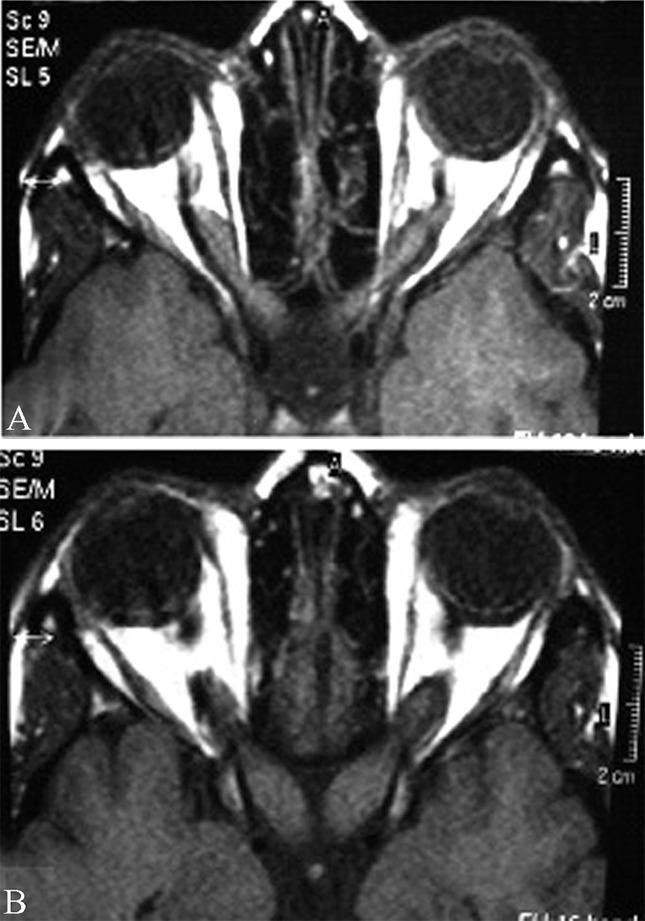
A 20-year old girl with progressive loss of vision and cafe-au-lait spots on her skin. A.Axial T1-weighted MR image of the orbits. B. Post-contrast axial T1-weighted MR image of the orbits.
